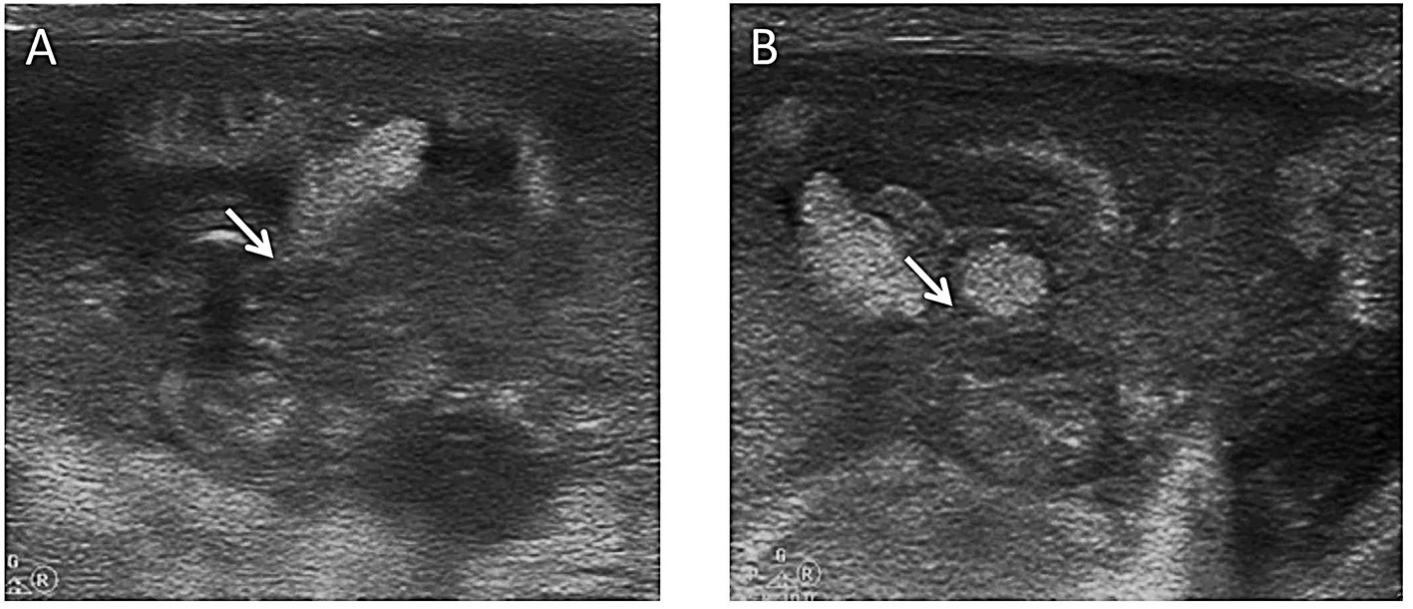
Location of perforation. An extra low birth weight boy (28 weeks of gestation) presented with abdominal distension on the 2nd day of life. Although medical therapy including fasting, antibiotics, and mechanical ventilation was given, the baby's condition kept worsening, and pneumoperitoneum was proven by an abdominal CT scan. He was transferred to our NICU for surgery. (A) Ultrasound showed that the continuity of the mid-abdominal small bowel wall was interrupted, and a hyperechoic mass (a fecal stone) was found at the perforation (arrow). (B) This image showed that the contents were flowing from the perforation in the bowel wall (arrow) ~10 min later.
